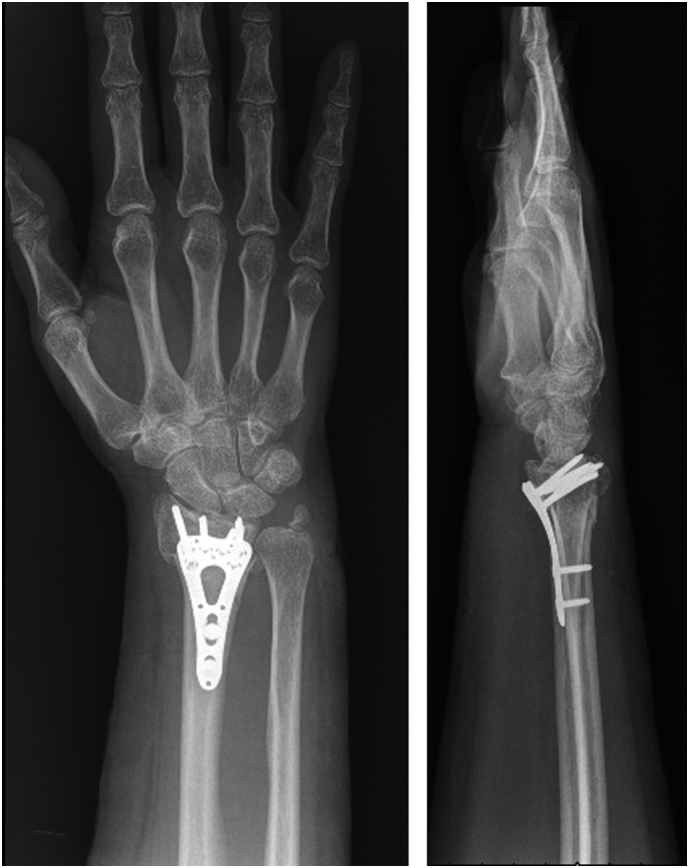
First postoperative radiographs demonstrating intact reduction.
